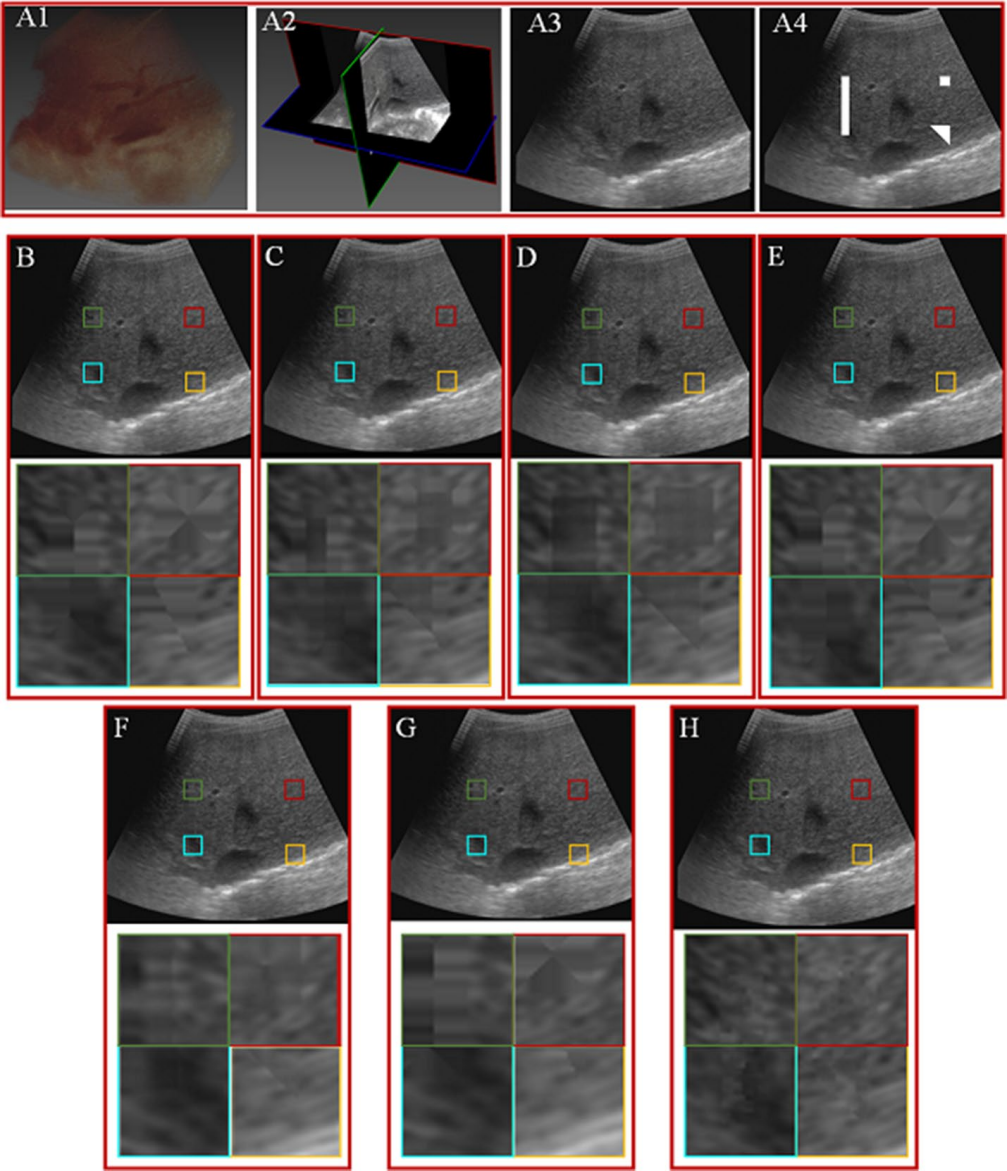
Comparison of different reconstruction methods over data 1. A1 3D rendering of ultrasound volume. A2 Cross-section of the ultrasound volume. A3 Ultrasound slice. A4 Ultrasound slice with holes. B–H Correspond to reconstruction results of the VNN, PNN, DW, FMM, KR, BI and the proposed GPM methods. The third column shows the magnified regions of interest corresponding to the second column
